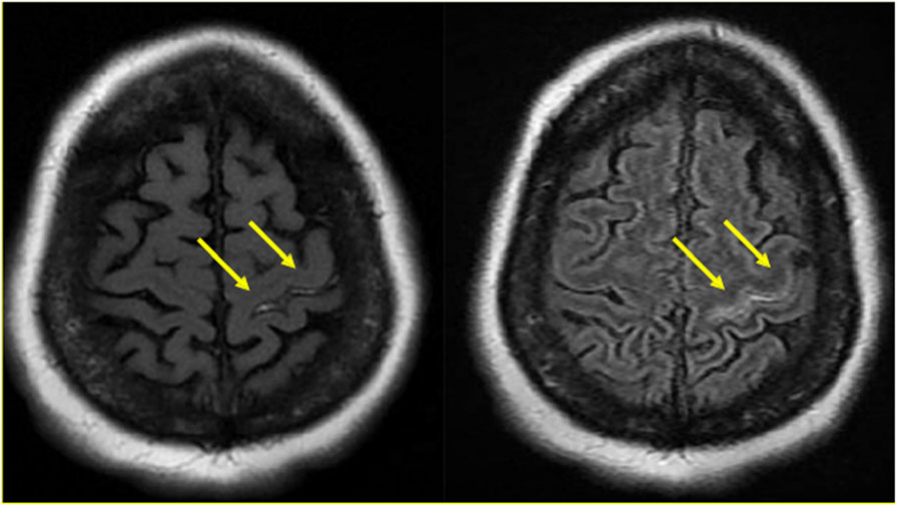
MRI of the brain demonstrating meningeal enhancement from pneumococcal dissemination
